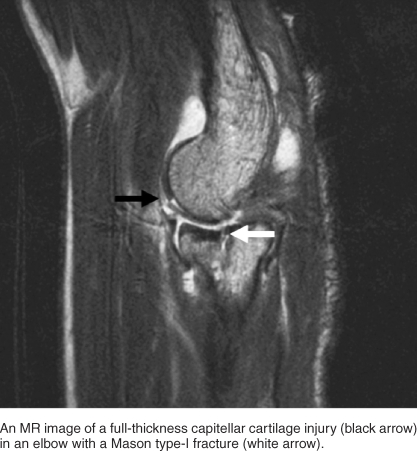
An MR image of a full-thickness capitellar cartilage injury (black arrow) in an elbow with a Mason type-I fracture (white arrow).
